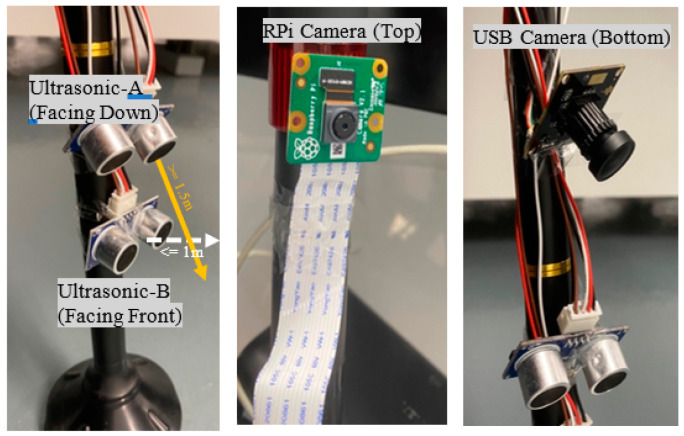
Ultrasonic (US) sensors and cameras.
